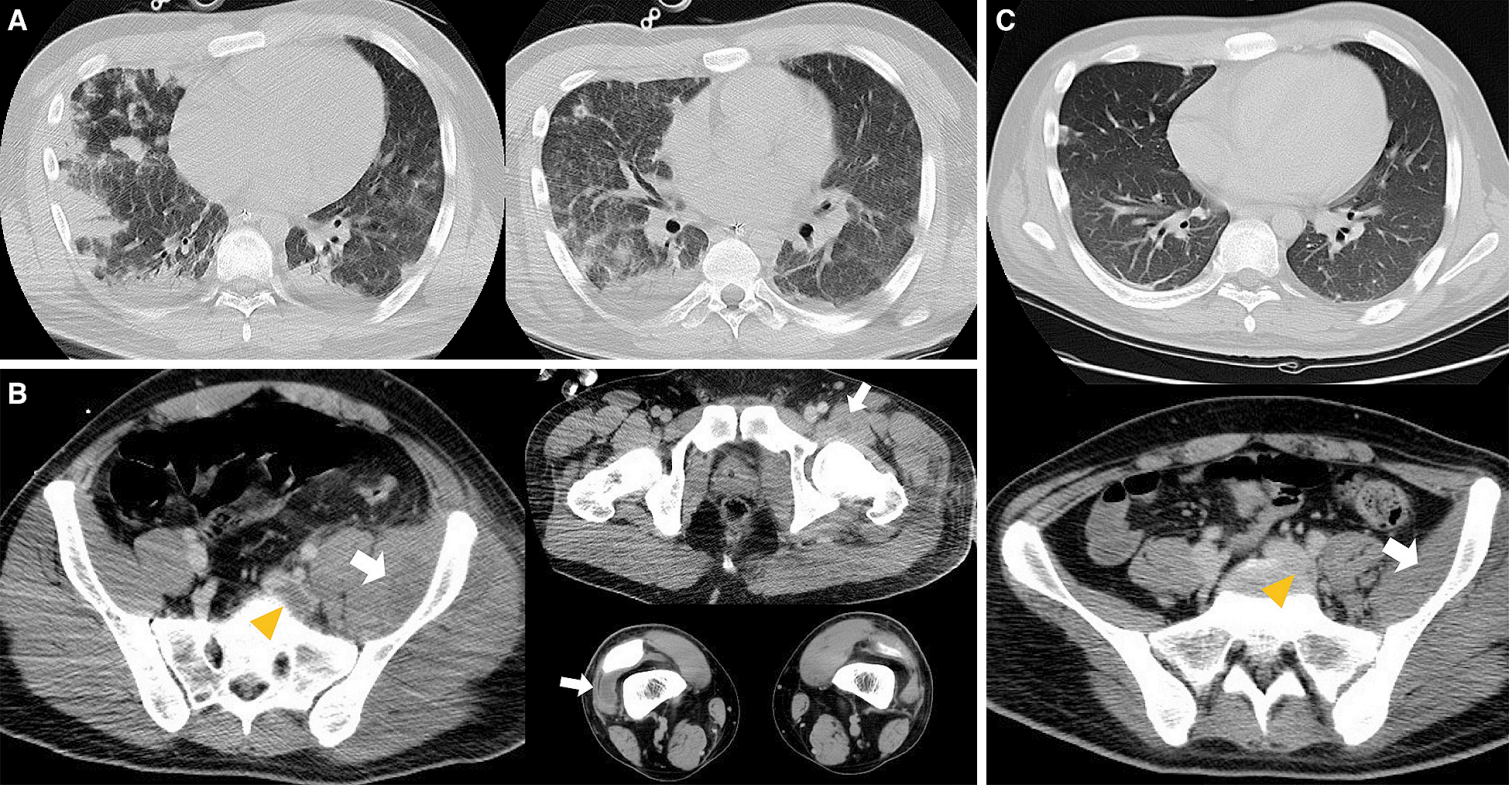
A) Contrast-enhanced computed tomography of the chest revealed septic pulmonary emboli. B) Contrast-enhanced computed tomography revealed left iliofemoral thrombosis (arrow head) and skin and soft tissue infection of the hip and left thigh with abscesses (arrow). C) CT after 1 month revealed the resolution of the iliofemoral deep venous thrombosis (arrow head).
